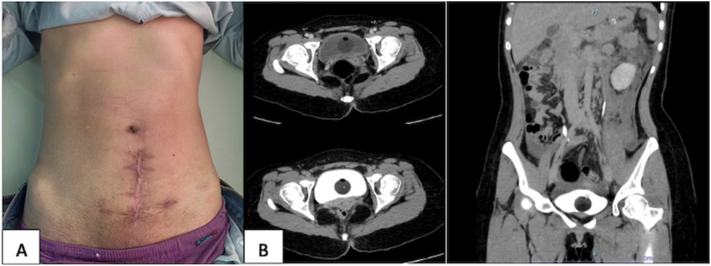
(A) Clinical Picture Post Operation Follow Up (B) CT Cystography Evaluation.
